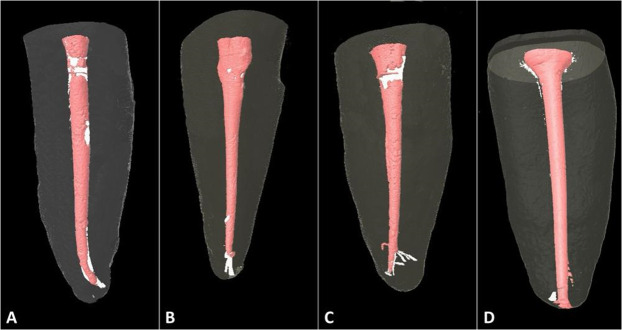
Three-dimensional computed tomography images displaying the filling of root canals by the root canal sealers. (A) MTA; (B) MTA-HA; (C) MTA-DCPD; (D) MTA Fillapex.
